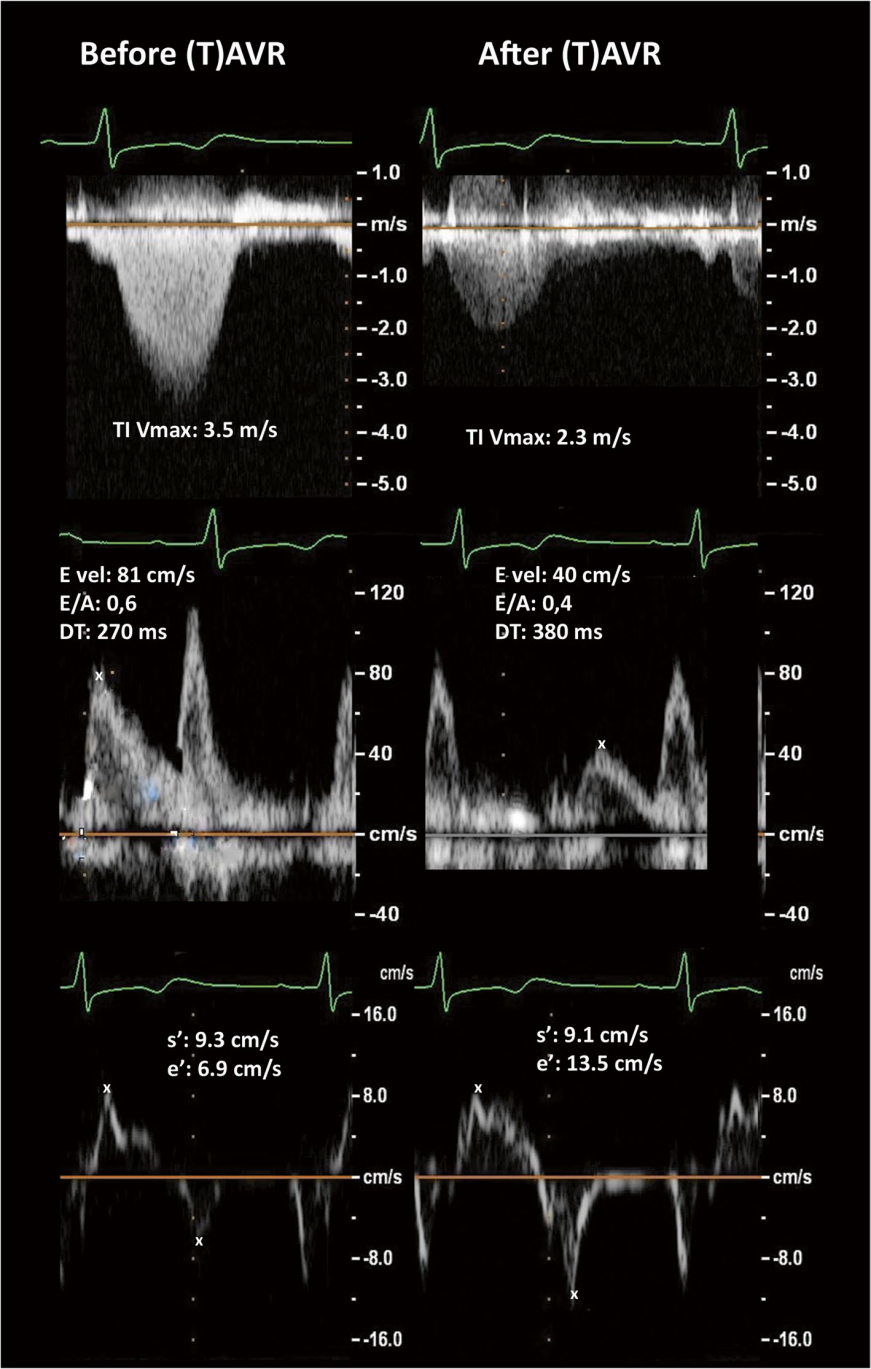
Example for mitral valve and tissue-Doppler parameters before and after aortic valve replacement (AVR); Upper panel: TI: tricuspid insufficiency; Vmax: maximal velocity; Mid panel: Mitral flow: E vel: E-wave velocity; E/A: ratio og E-wave and A-wave velocity; DT: deceleration time; Lower panel: Tissue Doppler: s’: peak systolic velocity; e’: peak e-velocity
